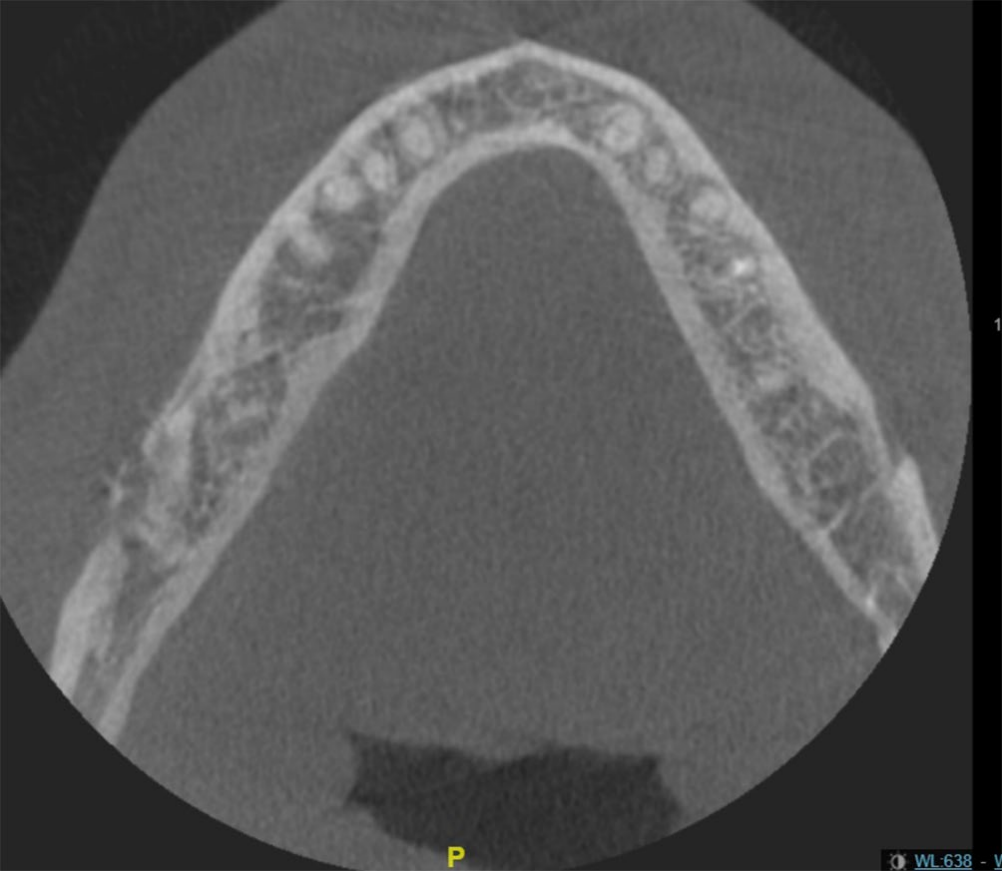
Axial view of Cone Beam CT: right mandibular osteomyelitis.
